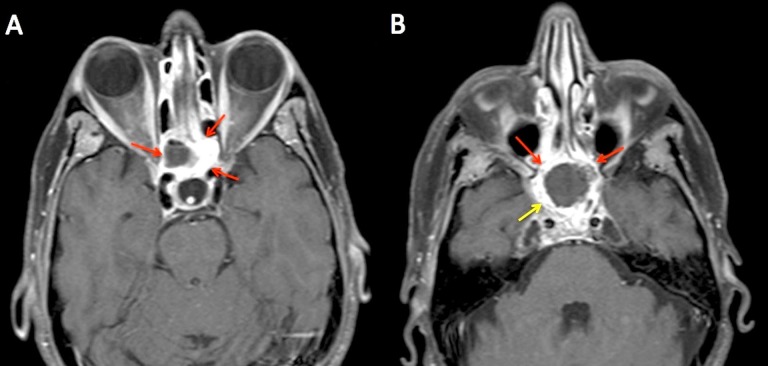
Representative axial slices of post-treatment MRI obtained at four months after completion of treatment Significant regression of the large nasopharyngeal mass and disease in the cavernous sinus (red and yellow arrows). Image A - the nasopharyngeal portion of the tumor had regressed significantly with residual cystic mass (red arrows). Image B - superior portion of the tumor was largely cystic (red arrows) and previous intracranial disease (yellow arrow) had regressed. MRI: Magnetic resonance imaging
